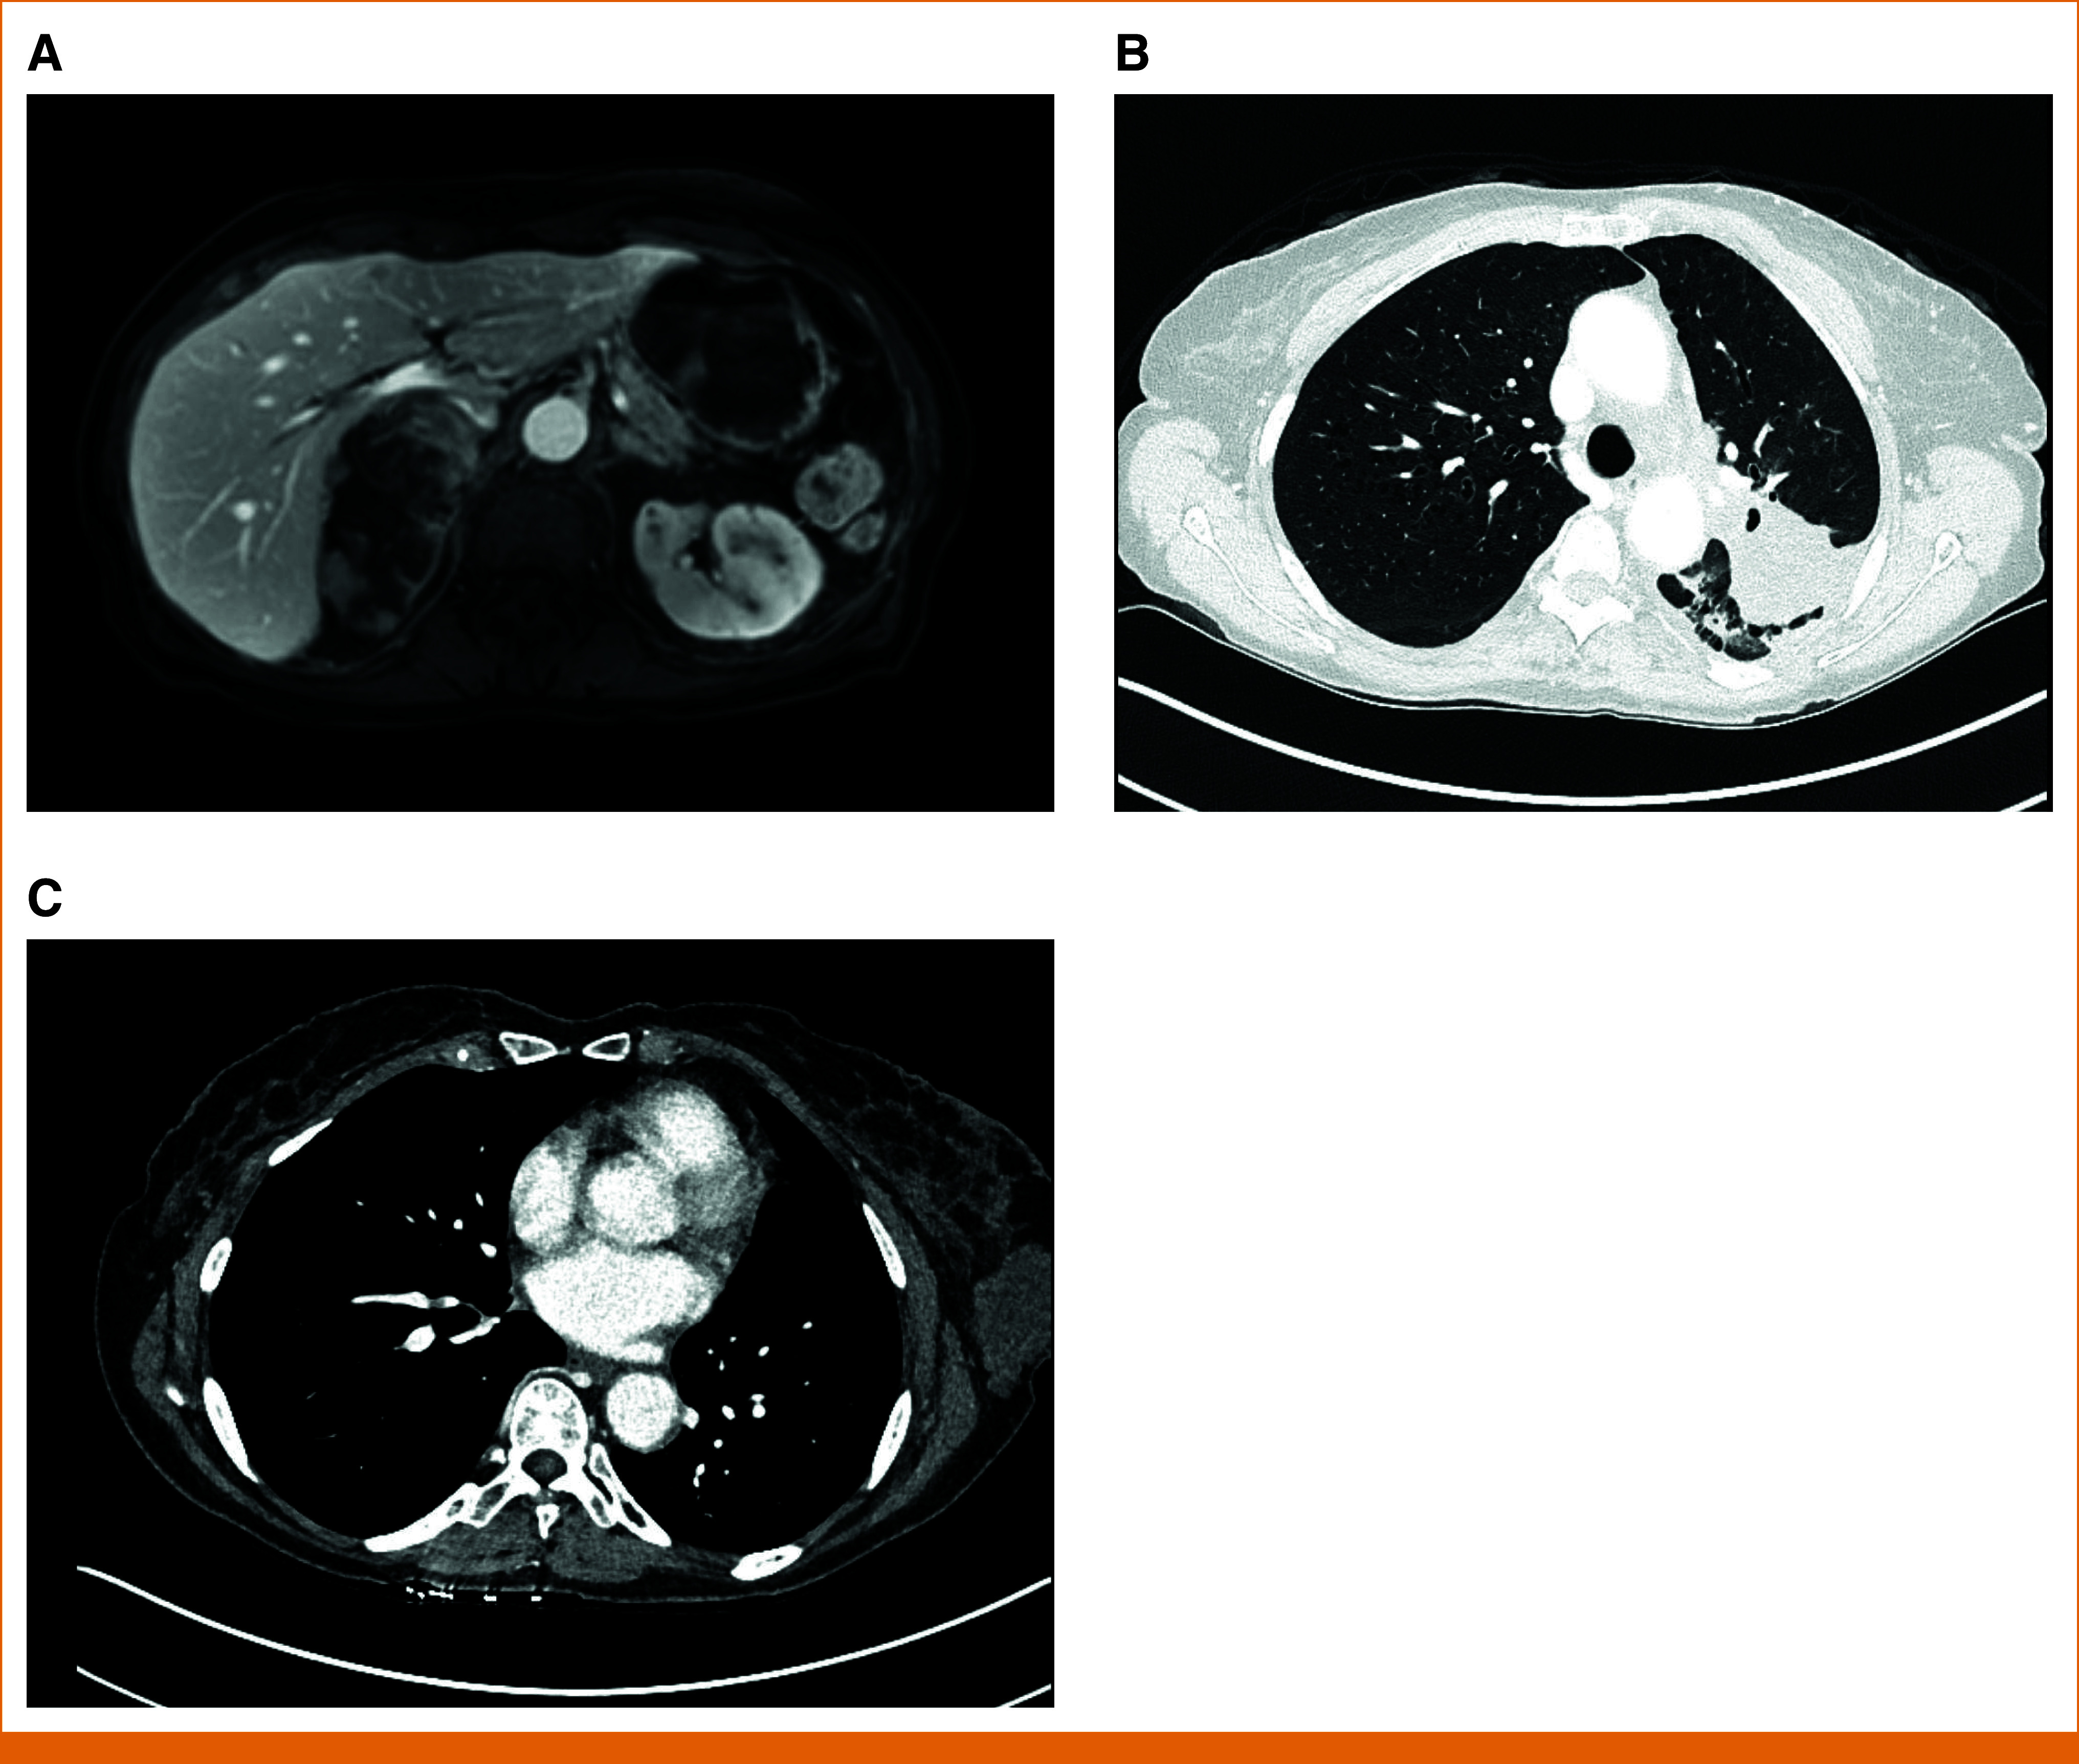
(A) Axial image from abdominal magnetic resonance imaging performed 6 months after treatment initiation shows a right adrenal mass with marked restricted diffusion and progressive hypoenhancement, measuring 8.5 × 5.1 cm. Mass effect on the adjacent right hepatic lobe, IVC, and liver hilum, as well as mild inferior displacement of the right kidney, is present. (B) Axial image from chest CT with contrast performed 6 months after treatment initiation shows the continuous decrease in size of the left upper lobe mass to 4.8 cm in largest dimension. (C) Axial image from chest CT with contrast performed 6 months after treatment initiation shows the continuous decrease in size of left breast mass to 51.9 mm in largest dimension. CT, computed tomography; IVC, inferior vena cava.
